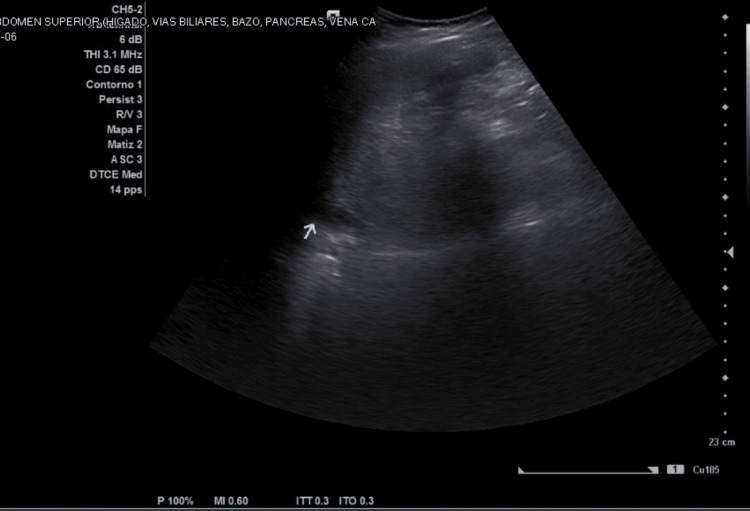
Abdominal ultrasound demonstrating perihepatic free fluid (arrow), consistent with plasma leakage during the critical phase of dengue infection.
